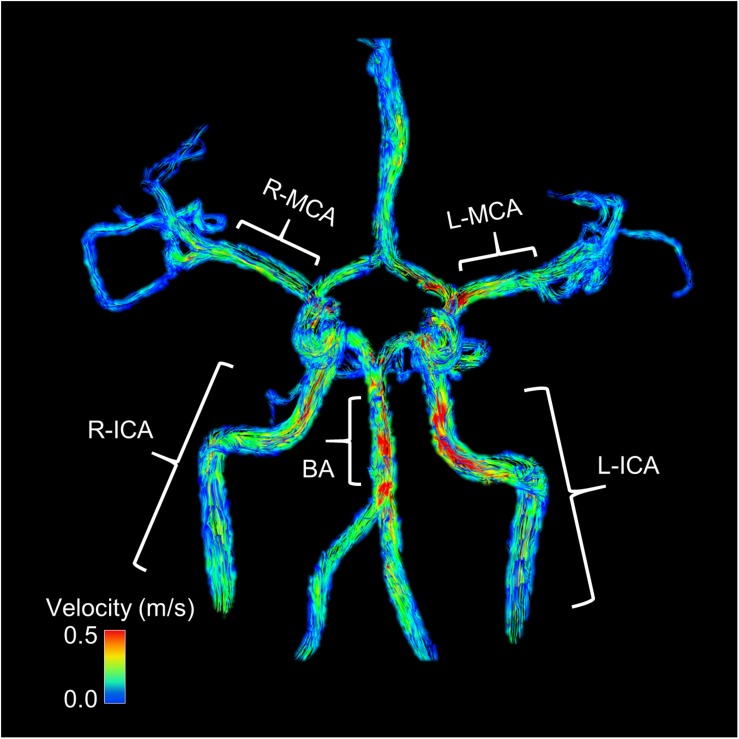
This image shows an example participant’s 4D flow MRI scan during normocapnia. Warmer colors indicate higher flow. Blood flow and vessel cross sectional area (CSA) were averaged along the length of the vessel. The middle cerebral arteries (MCA) were measured along the M1 segment. The internal carotid arteries (ICA) were measured along the cervical and petrous portions below the carotid siphon. The basilar artery (BA) was measured below the superior cerebellar artery and above the bifurcation of the vertebral arteries. Global flow was calculated as the sum of the right and left ICA and the BA flows. End points or branches were removed from the vessel analysis.
